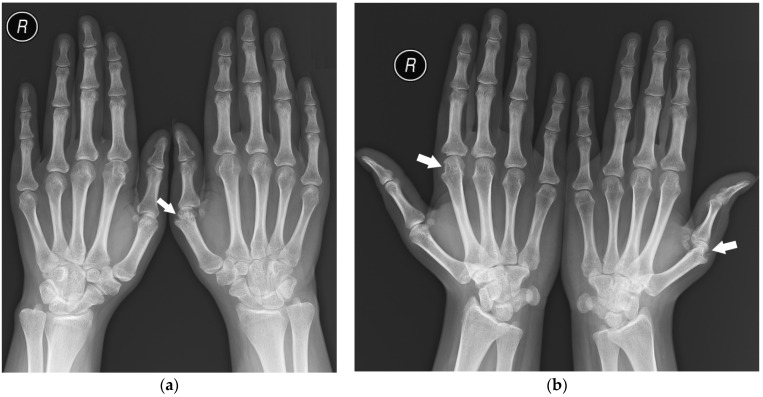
A 25-year-old female with seropositive RA. PA (a) and Nørgaard radiographs (b) of bilateral hands. Erosions, juxta-articular osteoporosis, joint space narrowing, and soft tissue swelling in several MCP joints, especially the left first MCP and right second MCP joints (arrows). Note the changes affecting the same compartments.
